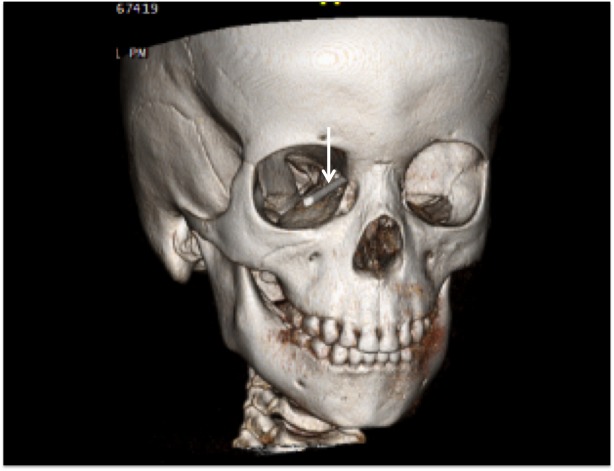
3D CT reconstruction of an adolescent who had fallen on a wooden stake (arrow). The stake entered the right orbit and traversed the ethmoid sinuses to become intracranial. 3D CT: three-dimensional computed tomography
